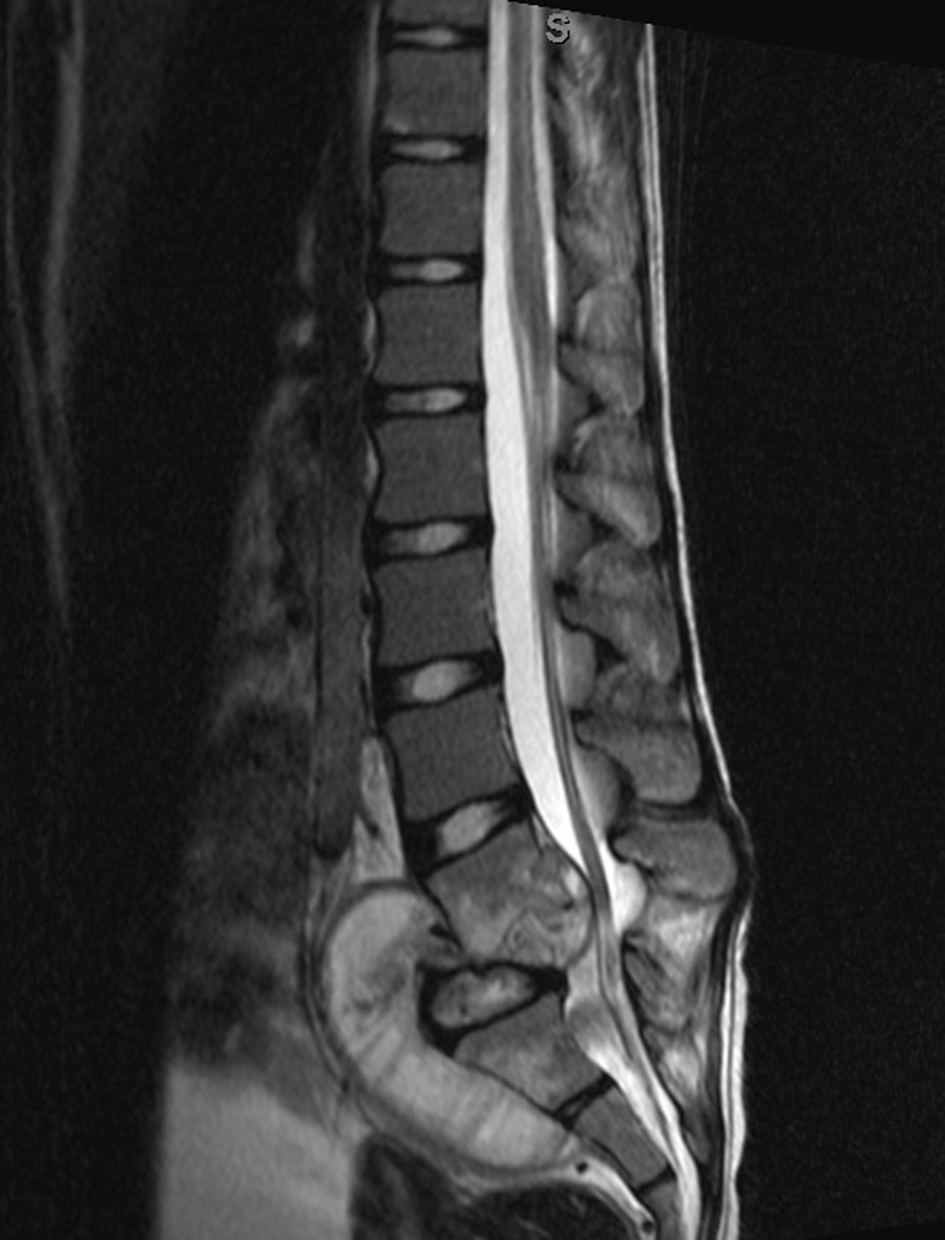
Sagittal MRI image of the lumbar spine of the same 12-year-old female with tuberculosis, showing multilevel involvement from L4 to S1 and a large anterior phlegmon
